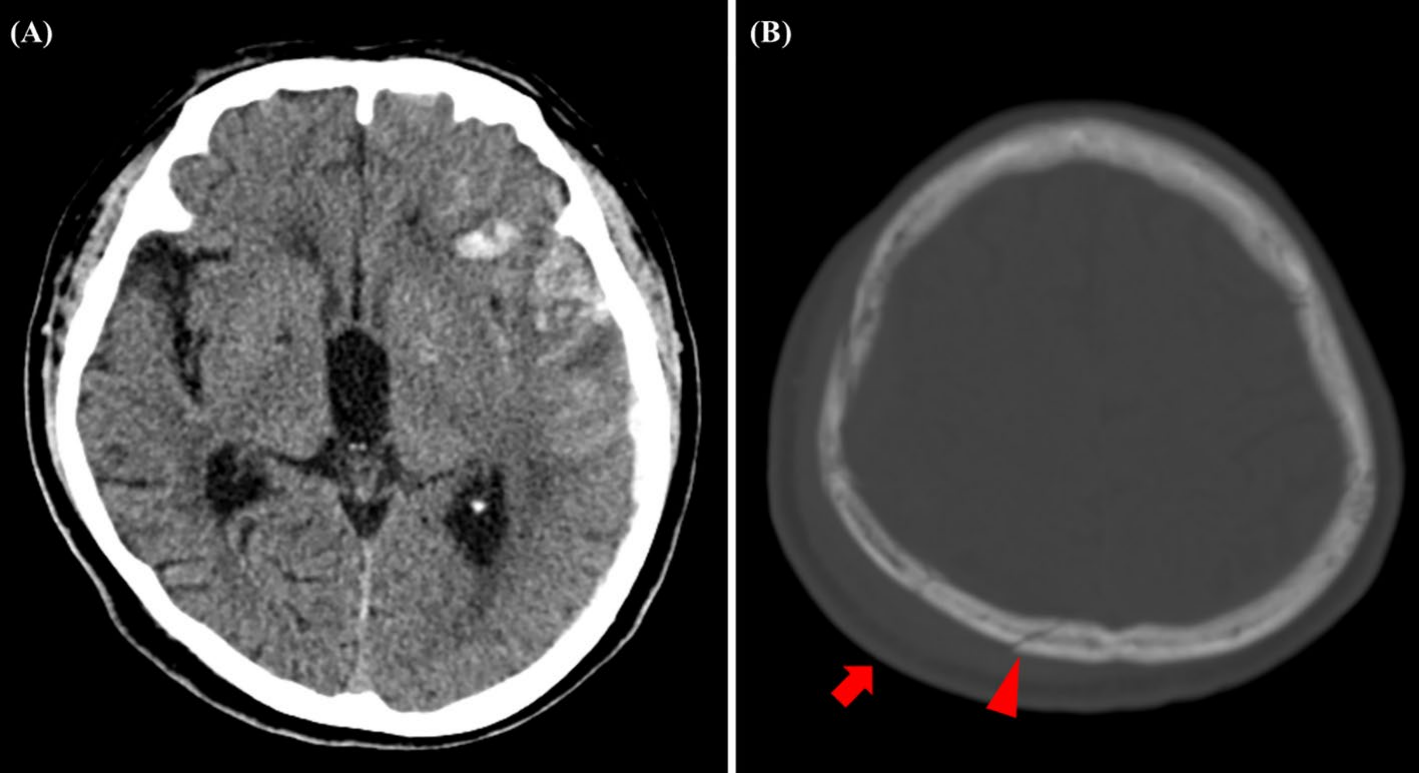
Counter Coup Intracerebral Hemorrhage (ICH) following Traumatic Brain Injury (TBI). (A) This CT scan depicts a case of counter coup ICH, where the hemorrhage is observed in the frontal lobe, opposite the site of initial impact, which in this case is the occipital region. (B) The corresponding bone window CT image shows a fracture in the occipital bone (indicated by red arrows), which is considered the site of the initial impact.
